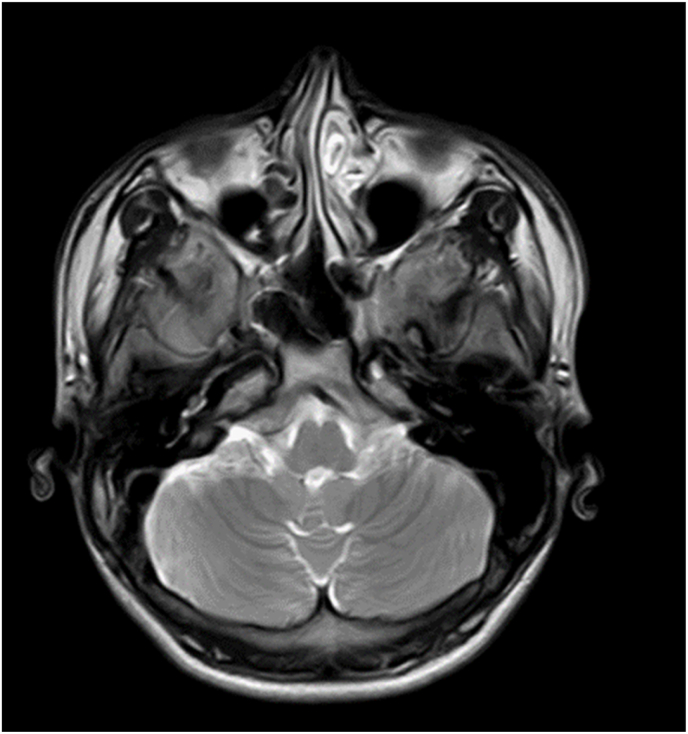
MRI follow up scan of cerebellum showing no altered signal intensity along the medial aspect of the bilateral cerebellar hemisphere.
